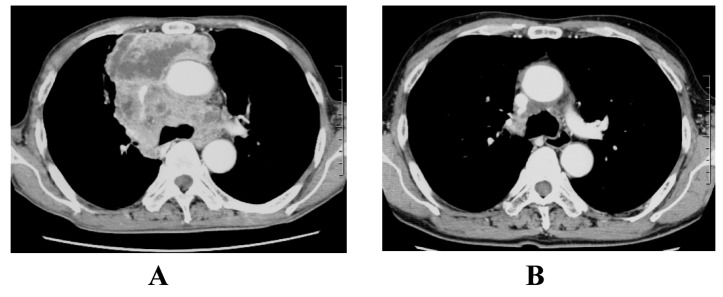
The response to initial ADOC chemotherapy in case 2. Chest computed tomography before initial ADOC chemotherapy (A) and after five cycles of initial ADOC chemotherapy (B).
